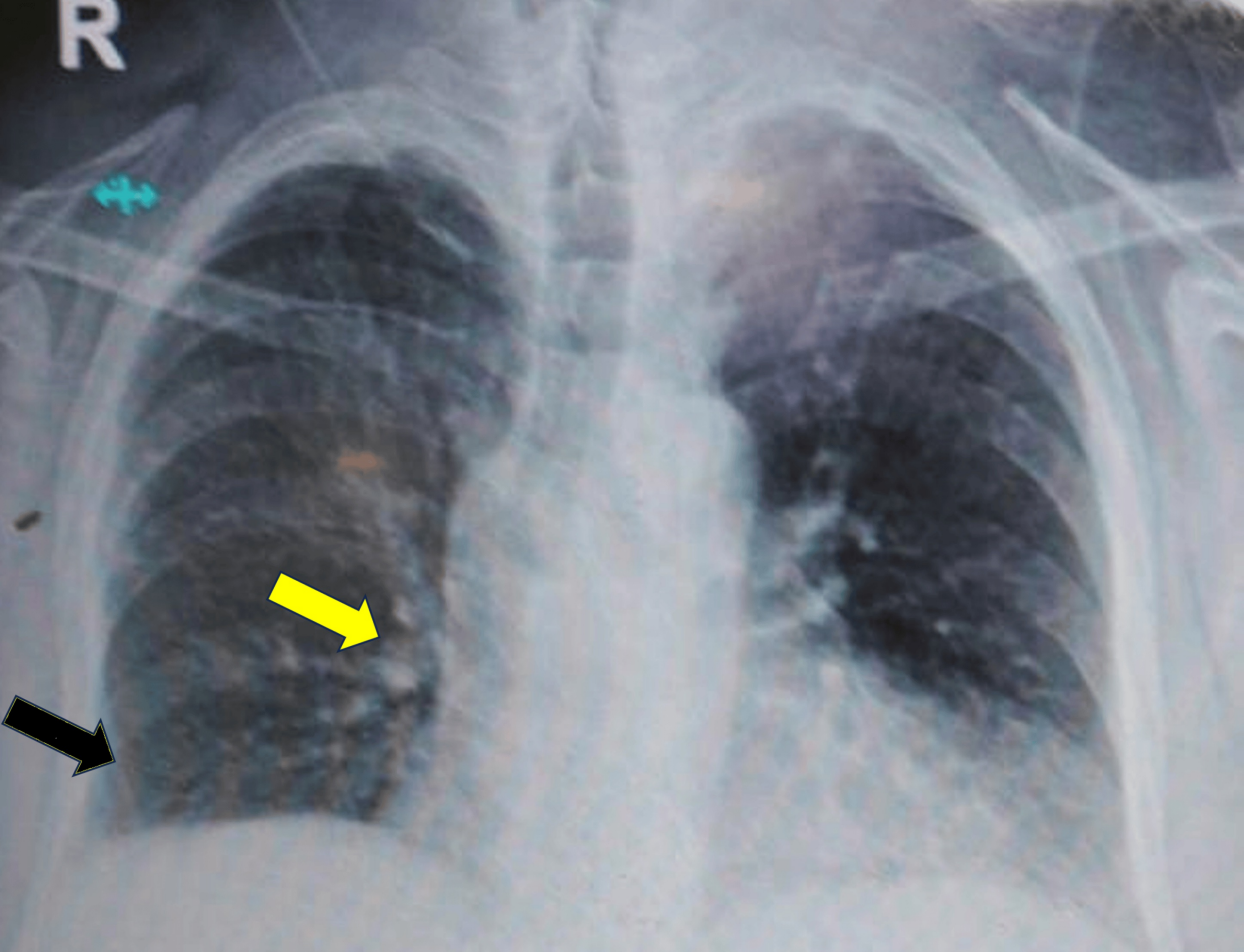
Chest X-ray showing minimal pleural effusion (black arrow) in the right basal zone and Palla's sign (yellow arrow), along with features of a lower respiratory tract infection Palla's sign: prominent enlargement of the right descending pulmonary artery.
